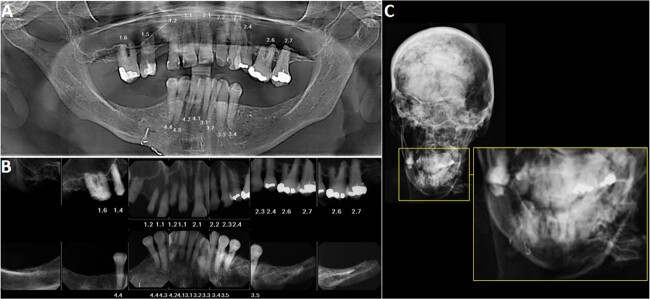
Radiographic images used for comparison in Case #1. (A) Antemortem panoramic radiograph, postmortem periapical radiographs. (B) Postmortem skull radiograph. (C) Detail of the skull radiograph showing the surgical wires (FDI/ISO dental numbering system).
